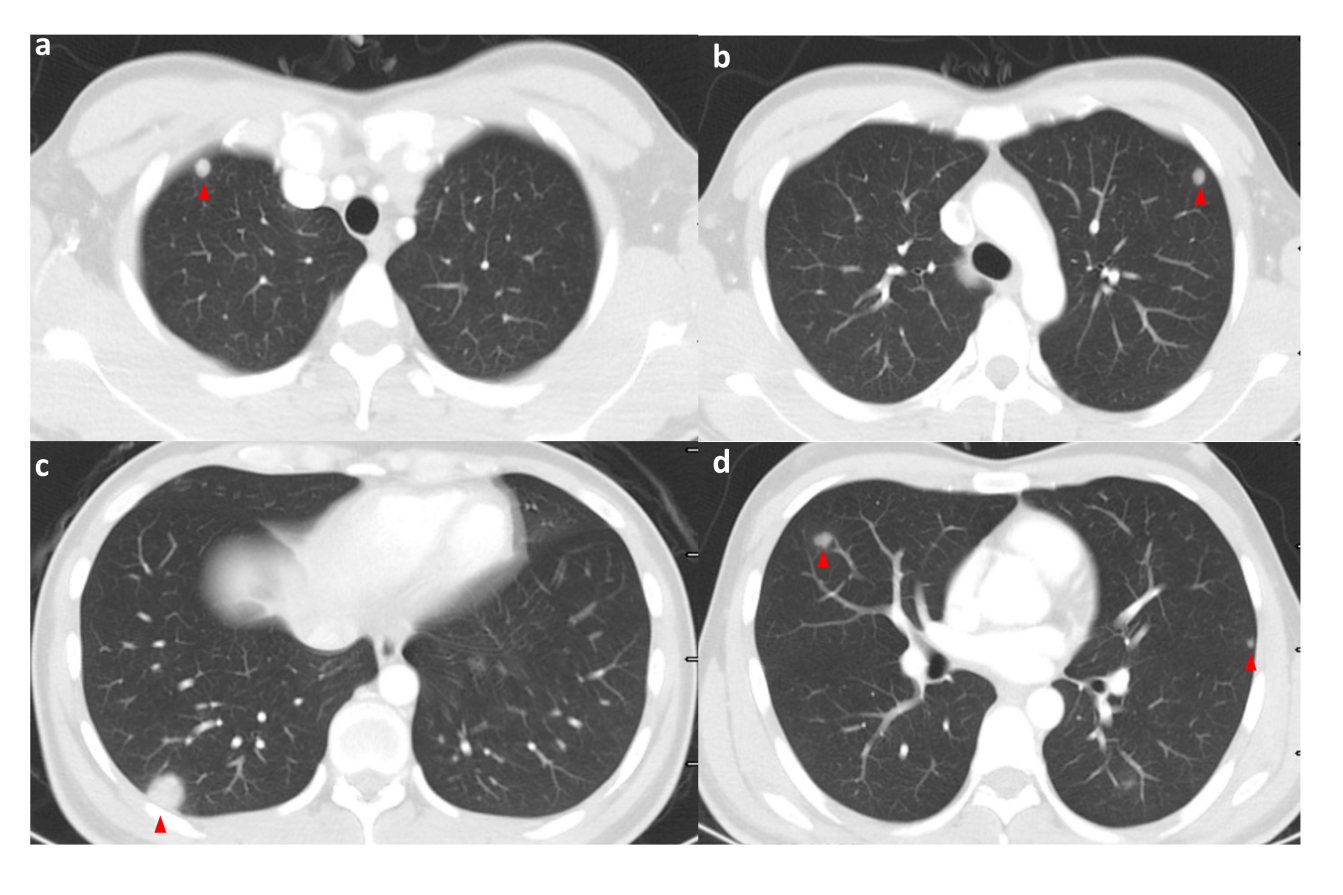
High-resolution CT of the patient on admission (pulmonary window); the patient’s lungs are seen to have multiple pulmonary metastases on admission. The red triangle points to the met. (A–D) shows high-resolution CT (lung window) images of different levels of the patient's lungs at the time of admission, where multiple pulmonary metastases were visible. Red triangles point to the metastases.
